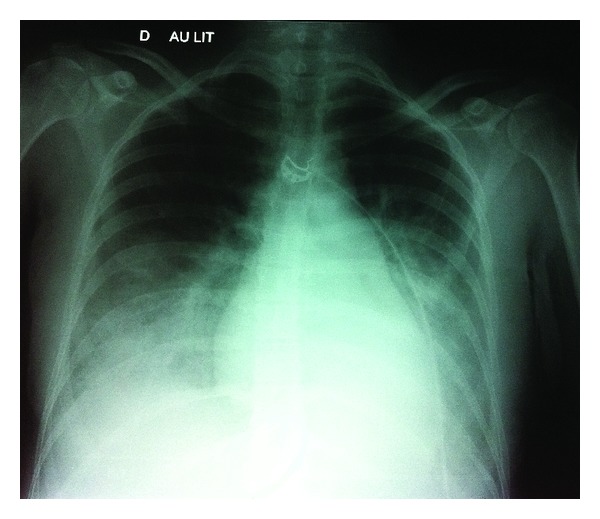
Chest X-ray face-on in prone position (Case 2).
